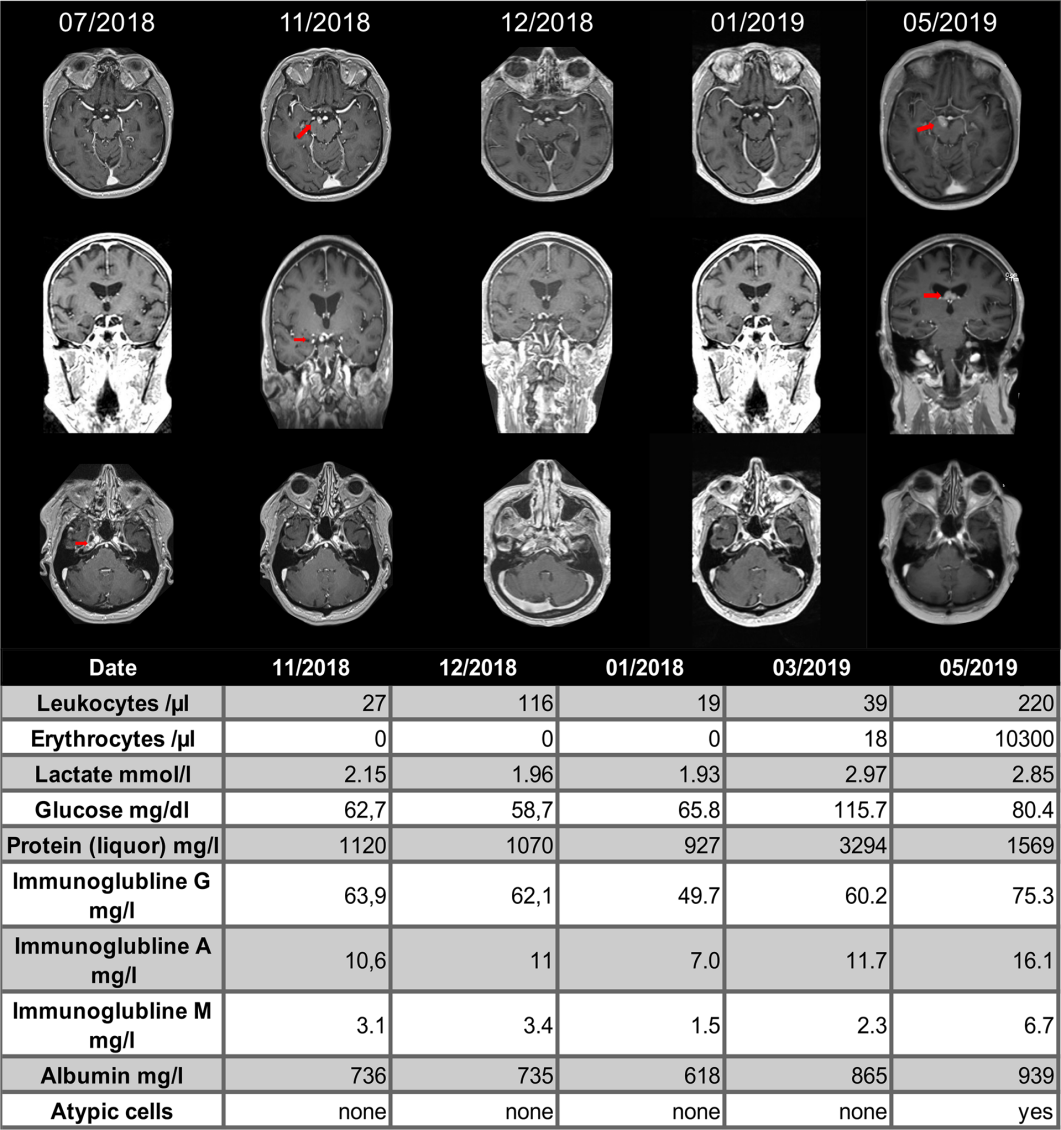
Cranial MRI scans of a 76-year-old patient with melanoma of the vulva. The patient had been treated with nivolumab and ipilimumab, which had been discontinued due to autoimmune hepatitis and switched to pembrolizumab in 03/2018. Routine staging revealed a new, contrast-enhancing tumor next to the right posterior cerebral artery (second column, red arrow). Repetitive cerebrospinal fluid analysis did not show meningeal carcinomatosis. Treatment with oral prednisolone was started in 12/2018 (third column) and the next MRI one month later showed shrinkage of the tumor, which was retrospectively diagnosed as pseudoprogression (fourth column). In 05/2019 the patient showed a meningeosis carcinomatosa in before normal appearing localizations.
